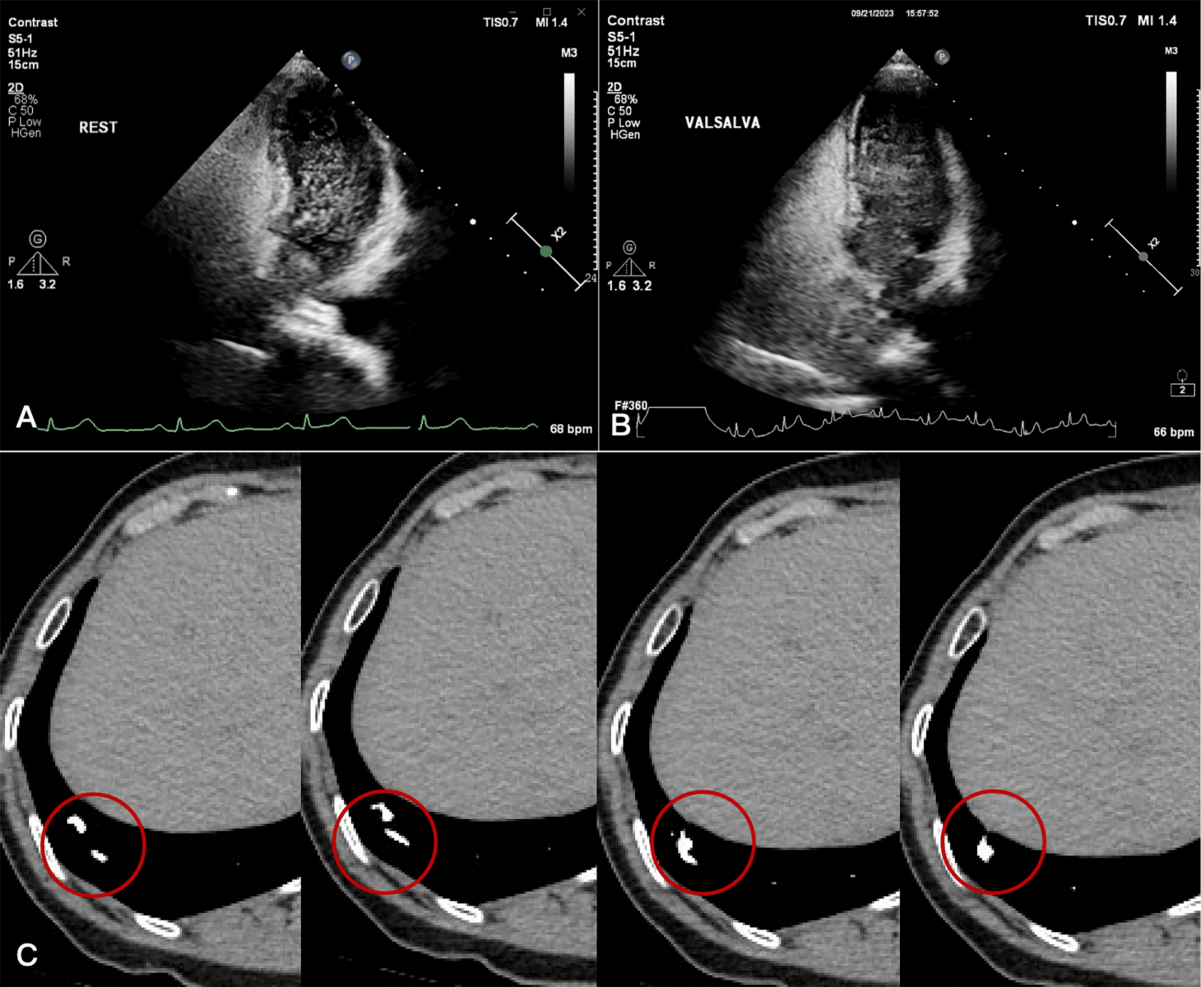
Transthoracic contrast echocardiography image and CT angiography of the chest. Transthoracic contrast echocardiography revealing a significant right-to-left shunt both at rest (A) and during the Valsalva maneuver (B). (C) CT angiography of the chest suggesting the formation of a pulmonary arteriovenous fistula in the lower lobe of the right lung (red circle).
